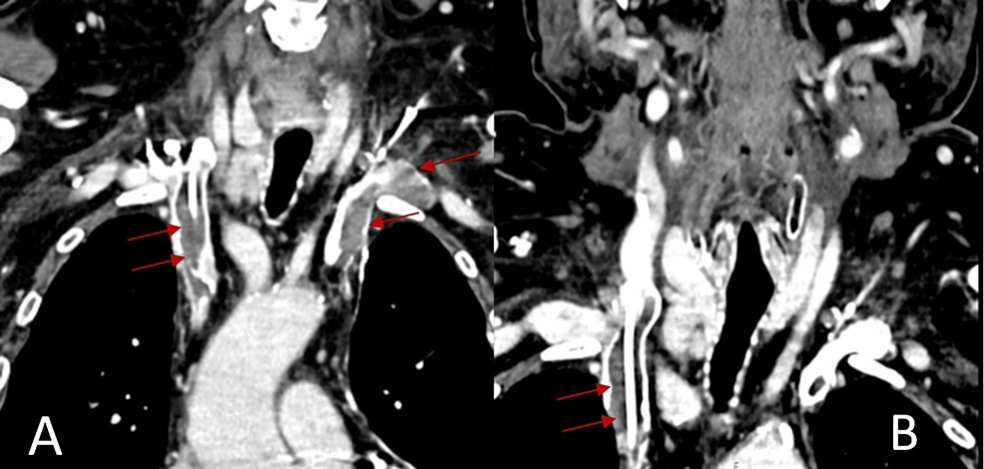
Thoracic (A) and cervical (B) angio-CT images showing thrombi in the superior vena cava and left subclavian and right internal jugular veins, as well as a right jugular central venous catheter (red arrows).
